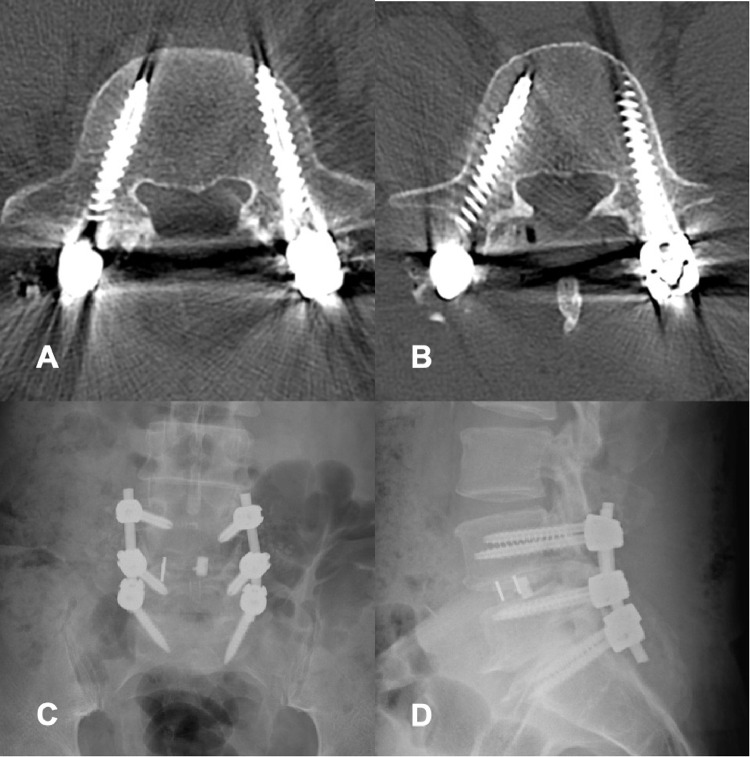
Postoperative images: (A) axial CT at L5 and (B) axial CT at L4 showing adequate position of navigated screws without pedicle perforation, (C) antero-posterior and (D) lateral radiographs showing final spinal fusion construct.
